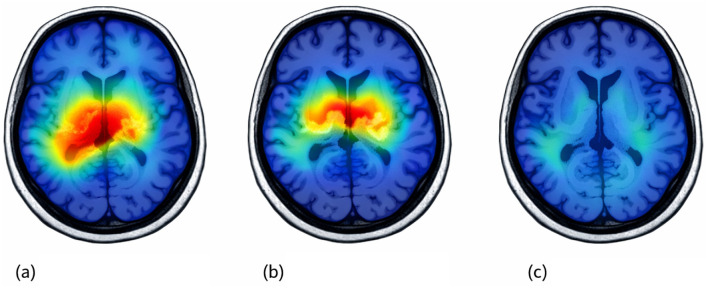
Grad-CAM++ visualizations on brain MRI images for (a) Alzheimer's disease, (b) Parkinson's disease, and (c) healthy control, highlighting regions important for model prediction.
